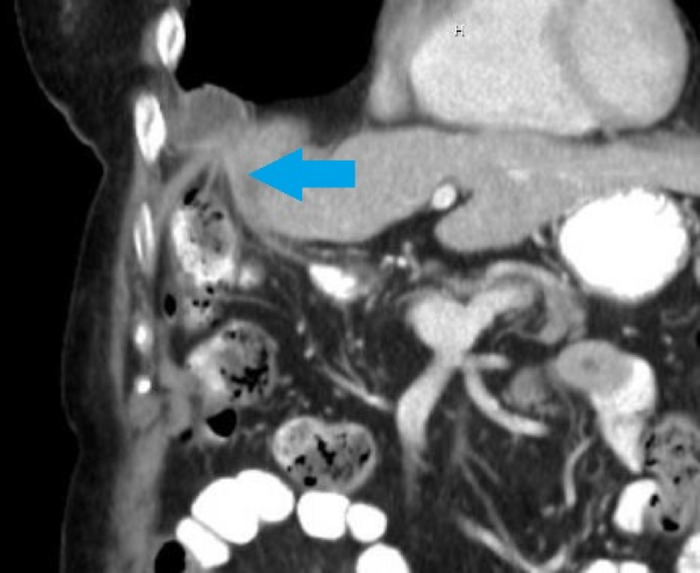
Computed tomography coronal view shows gallbladder diaphragmatic herniation with prominent cystic duct (arrow).
